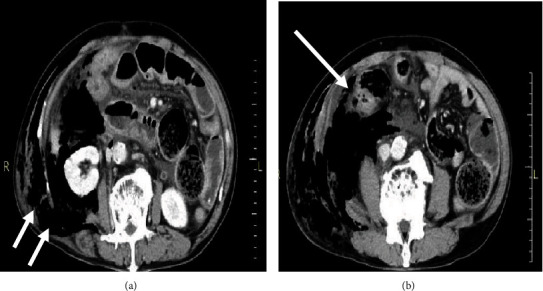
Radiological findings: (a) abdominal contrast-enhanced computed tomography (CT) scan showing fluid collection, an air pocket in the subcutaneous fat layer of the abdominal wall, and edematous changes in the intraperitoneal and abdominal wall adipose tissue (arrow); (b) inflammatory changes are observed around the ascending colon (large arrow).
